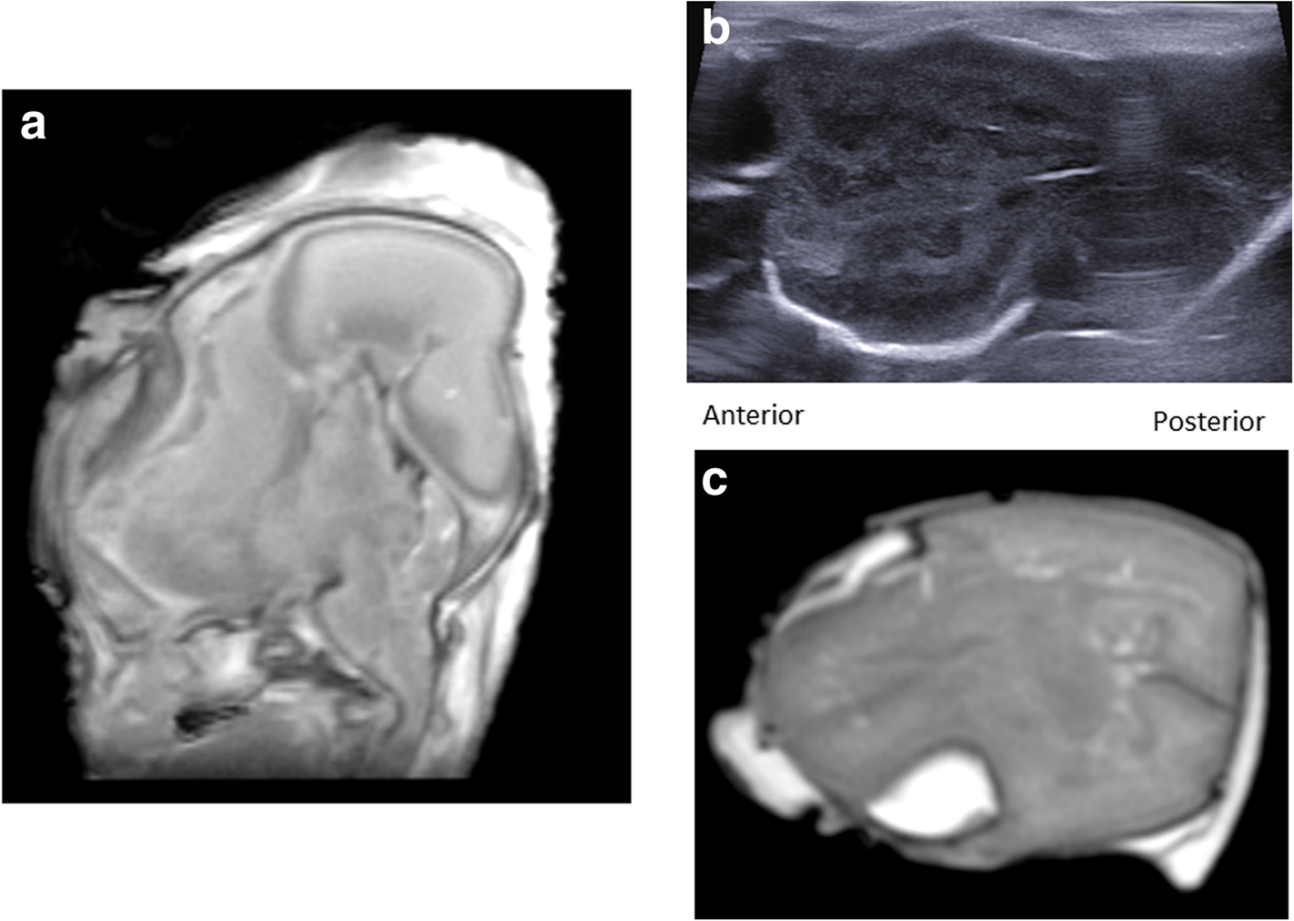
Images of delivery and maceration related changes that degrade postmortem ultrasound image quality in a 22-week gestational-aged foetus. Sagittal T2-weighted postmortem MRI at 1.5 T (a) through the midline shows soft tissue oedema of the scalp, overlapping cranial sutures and underlying brain maceration. On the corresponding left trans-temporal view of the brain at postmortem ultrasound (b) and at axial postmortem MRI (c) at the level of the midbrain, it is difficult to delineate normal brain parenchymal architecture. In such cases, the imaging is described as ‘non-diagnostic’ and useful information cannot be gleaned by either imaging modality
